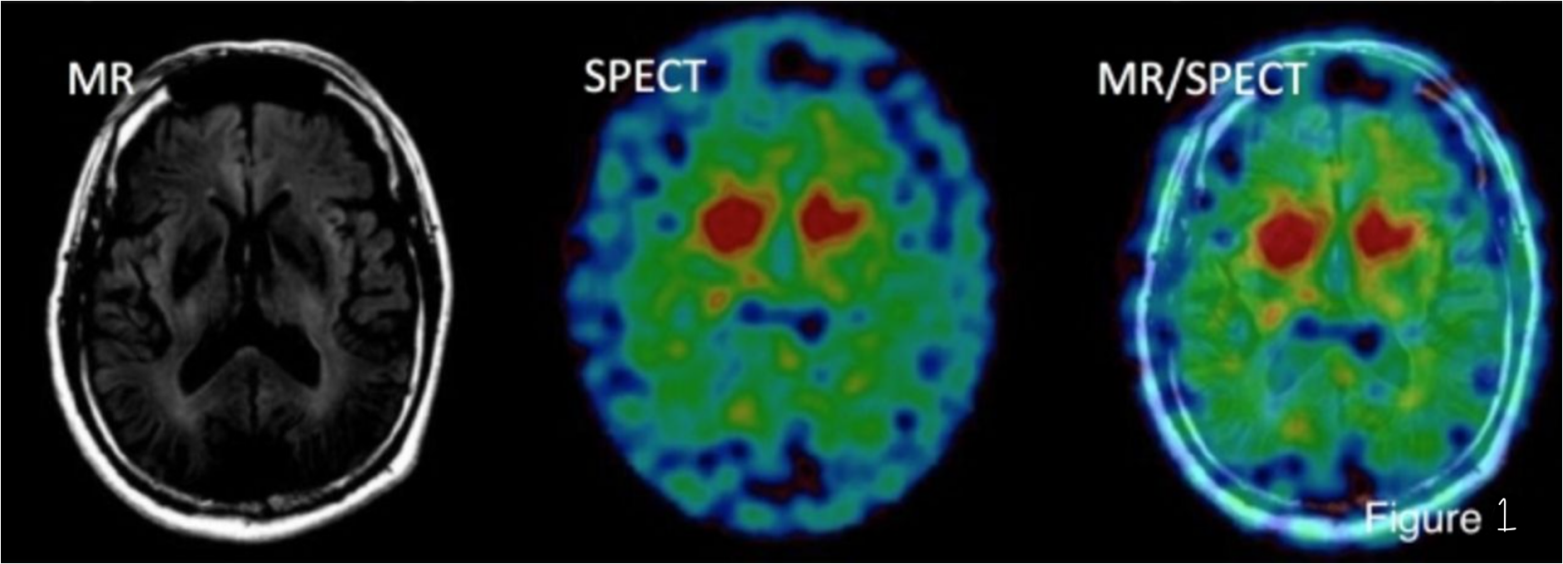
MR and SPECT images have been fused to create a MR/SPECT image. Adapted from Takeshi Hara et al. (2016) J Nucl Med. 57:1936.
